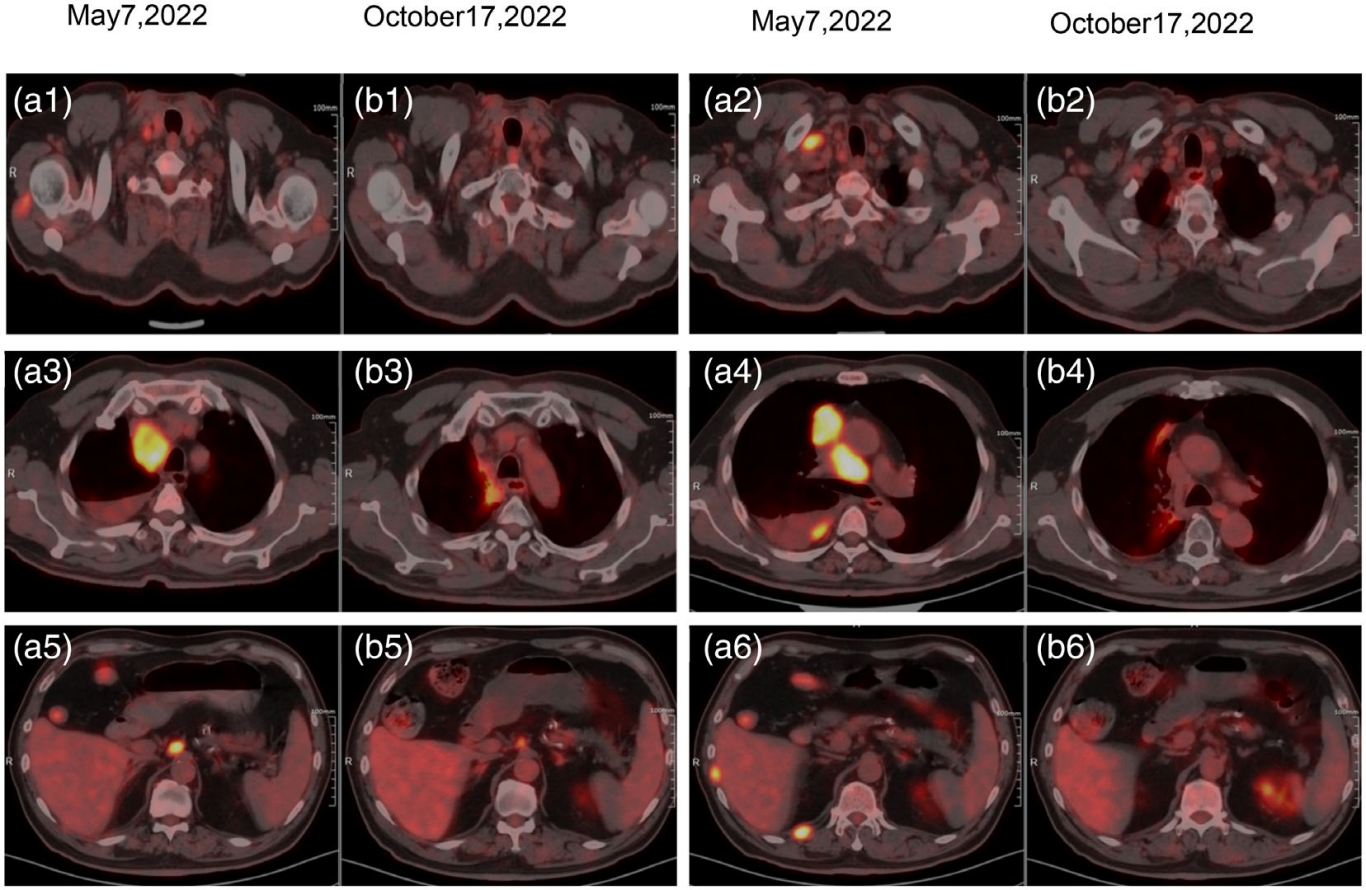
Comparison of ^18^F‐fluorodeoxyglucose positron emission tomography/computed tomography (^18^F‐FDG PET‐CT) images after 5 months following PD‐L1‐enhanced exogenous pericytes combined with antiangiogenic targeting therapy. (a1–a6) The patient's pretreatment images from May 7, 2022. (b1–b6) The patient's images from October 17, 2022.
